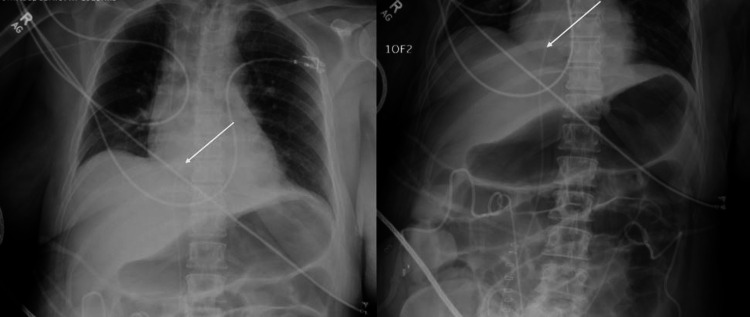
AP chest X-ray (left); KUB (right) AP chest X-ray (left): demonstrating a nephroureteral stent (white arrow) in the IVC extending into the right atrium. This was read as a thin catheter projecting over the right side of the abdomen and in the mediastinum. KUB (right): demonstrating a right nephroureteral stent (white arrow) in the IVC extending into the cardiac silhouette. This was read as bilateral nephrostomy tubes and ureteral stents in situ along with right iliac stent and right internal iliac coils. AP: anteroposterior; KUB: kidney, ureter, and bladder X-ray; IVC: inferior vena cava
